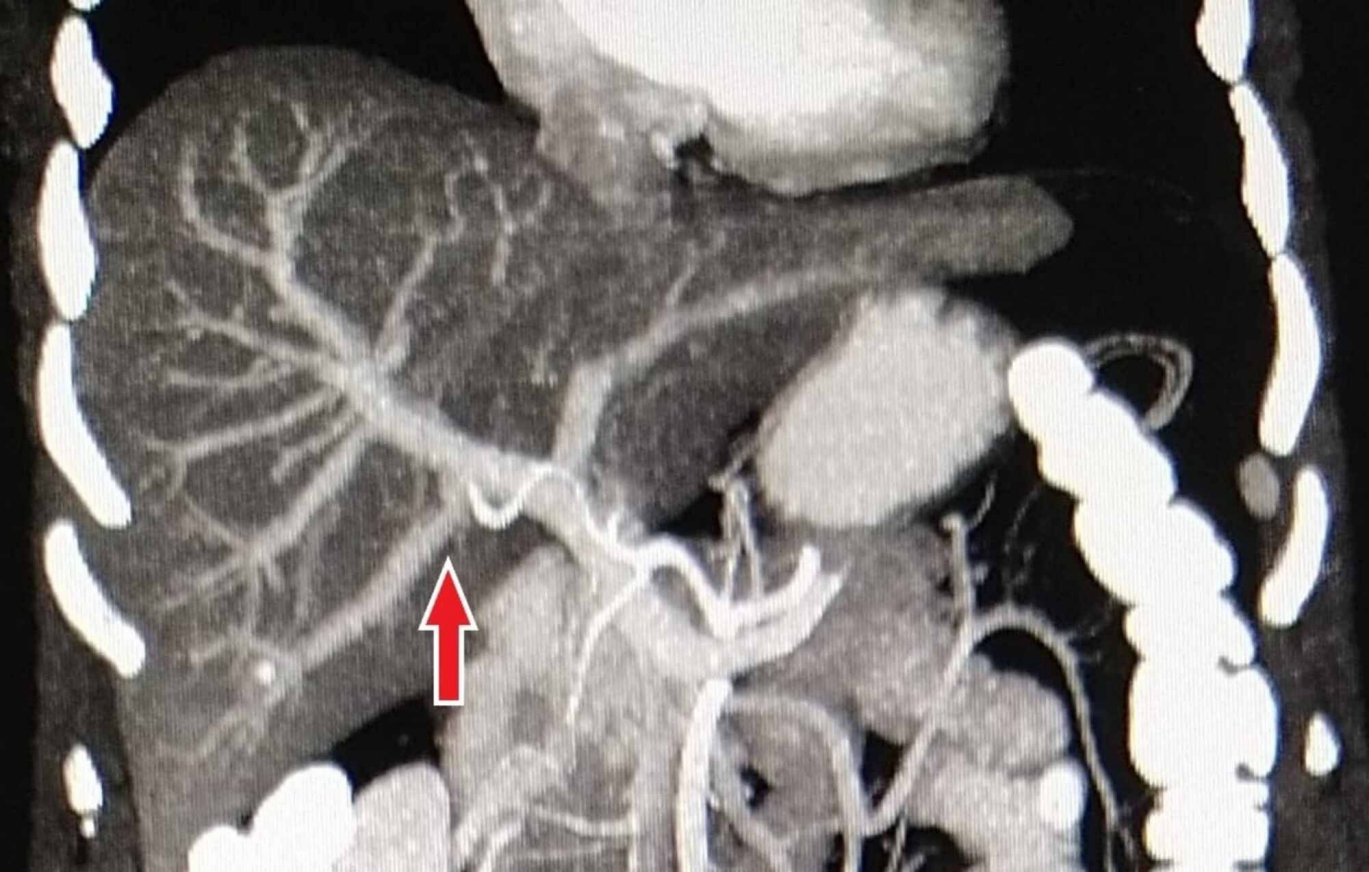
Contrast-enhanced CT MIP reconstructed coronal image showing the separate branch of the right portal vein to segment VI (type 5) MIP, maximum intensity projection.
